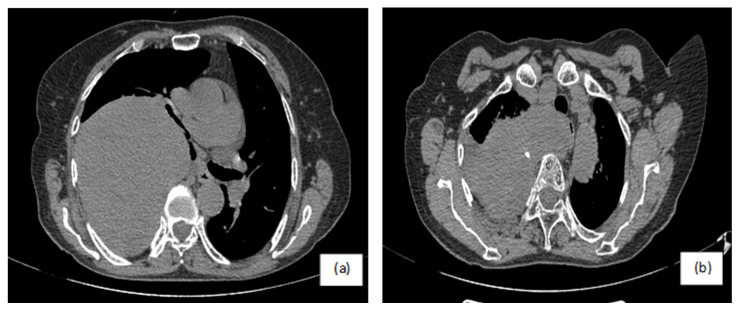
Initial chest CT from 10 years ago. (a) Large tumoral mass causing right main bronchus compression and mediastinal displacement toward the contralateral side; (b) focal calcification at the superior pole of the tumor with compressive effect on the esophagus. A small, loculated right-sided pleural effusion is also present. No evidence of mediastinal lymphadenopathy. Source: authors’ archive.
